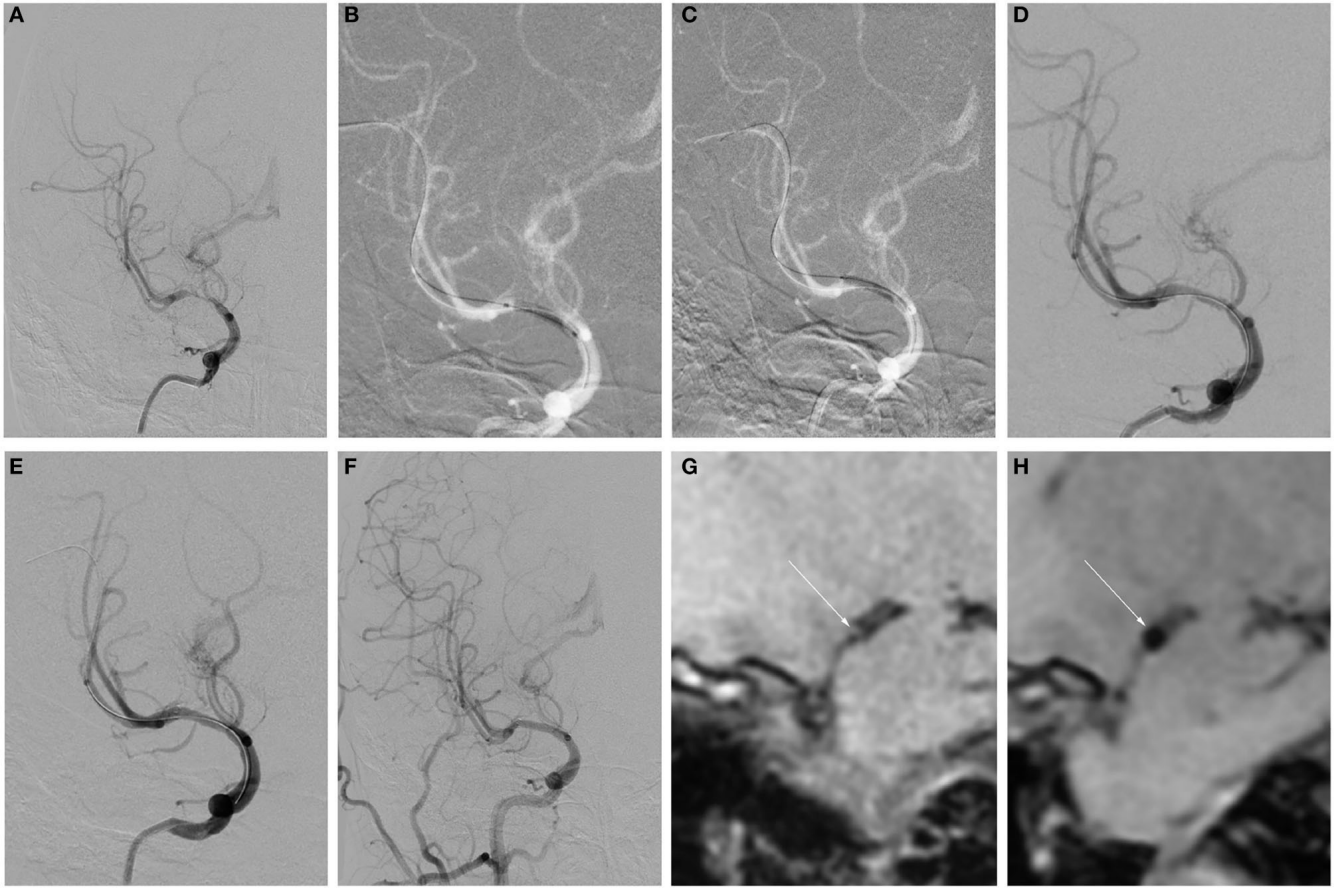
Example of remedial stent implantation after DCB dilatation and follow-up. (A–H) Angiography and HRMRI results of remedial stent implantation after DCB dilatation during the procedure and follow-up. (A) Severe right MCA stenosis. (B) Predilatation with a conventional balloon. (C) DCB dilatation after predilatation. (D) The angiographic result after DCB. (E) The angiographic result after remedial stent implantation. (F) Angiographic result at 5.5-month follow-up. (G) Focal eccentric plaque identified at target vessel wall [arrow in (G)] in high-resolution T1-weighted imaging sequence. (H) Slight circumferential wall thickening was identified on a high-resolution T1-weighted imaging sequence at a 5.5-month follow-up.
